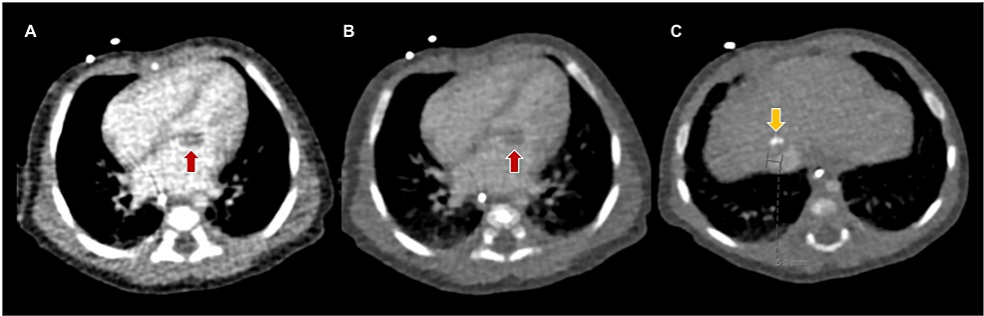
Cardiac and abdominal computed tomography image. Cardiac (A and B) and abdominal (C) computed tomography images show masses in both the heart (red arrow) and liver (yellow arrow), which exhibit very similar imaging characteristics: both are vascularized with intense contrast agent uptake and both contain calcification foci.
